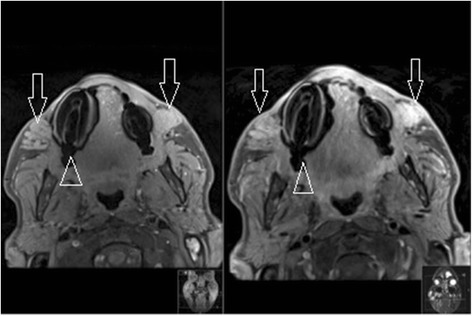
Polyacrylamide gel axial dermal filler appearance in both infraorbital triangles (*open arrows*) on axial MRI (T1FS, T1 FS with gadolinium). Note the susceptibility artifacts from dental implants (*open arrowhead*) while the filler’s signal intensity remains high before and after injection of gadolinium
